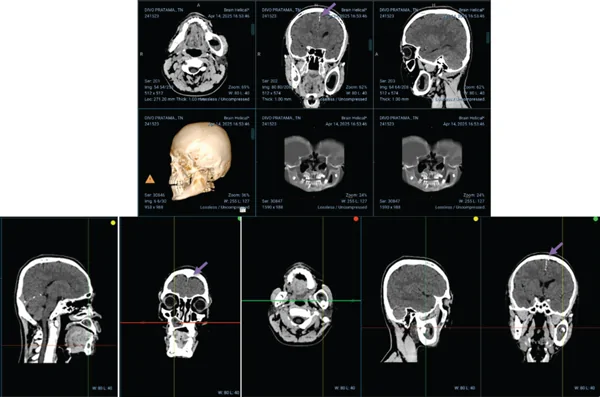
CT scan showing bilateral calcification of the falx cerebri.
